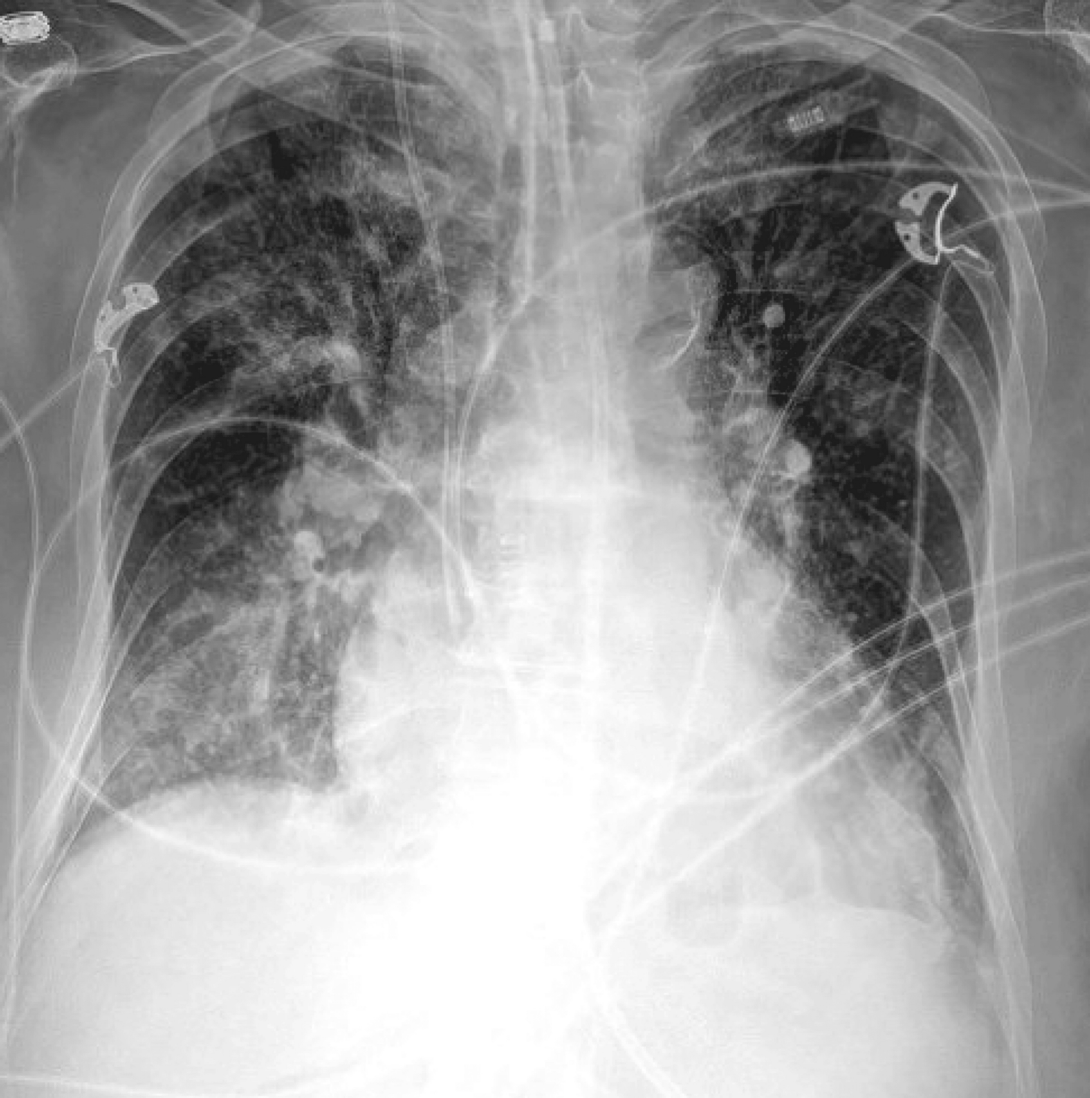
Chest radiograph on day 10 of hospitalization showed progression of bilateral infiltrates with worsening pulmonary edema.
